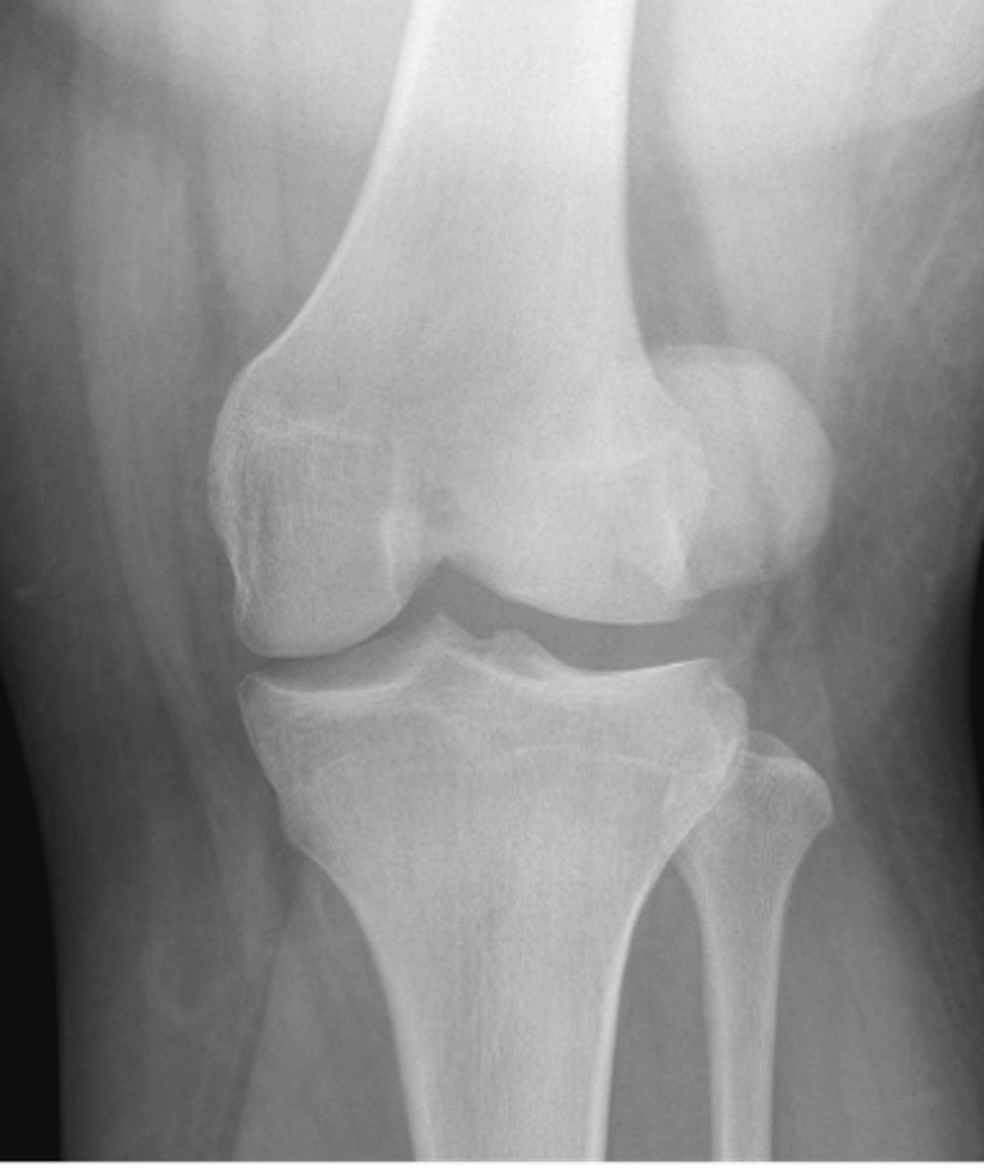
Anterior-posterior radiograph of the left knee showing a lateral patellar dislocation
